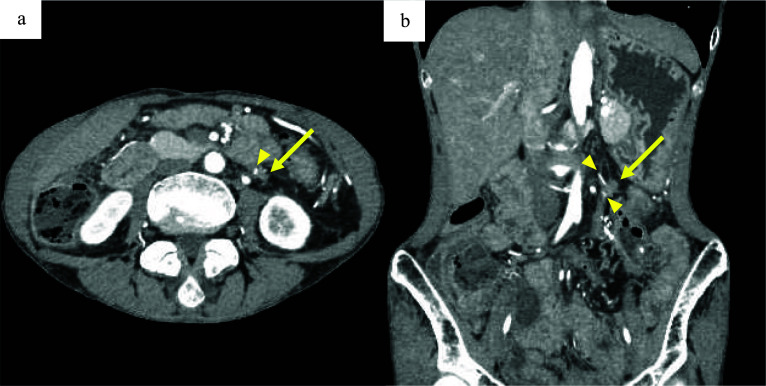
Follow-up preoperative contrast-enhanced computed tomography (CT). Preoperative contrast-enhanced CT shows an enlarged mass (arrow) with embedded clips (arrowheads). a Axial view of the 15 mm mass, 5 mm larger than that seen in the initial follow-up CT, performed 3 months before. b Coronal view of the mass adjacent to the inferior mesenteric artery
